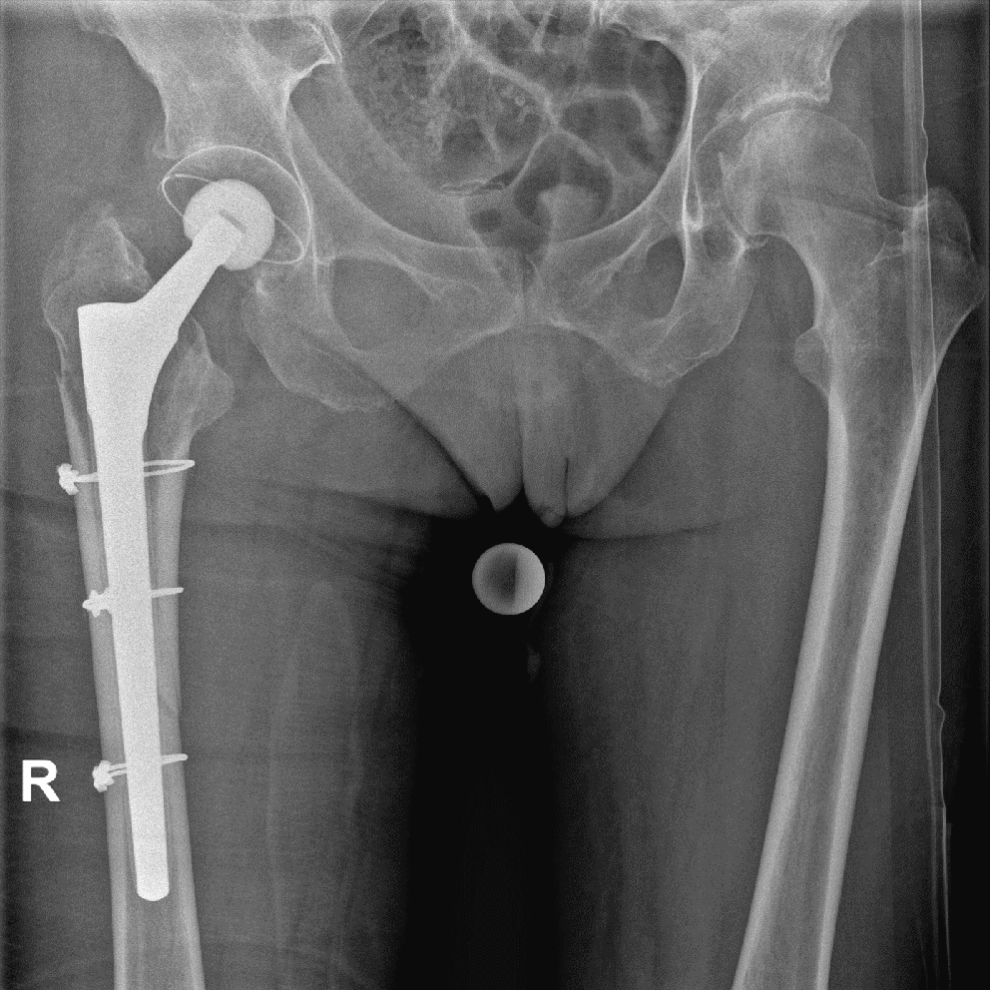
Postoperative Radiograph of the Vancouver B2 fracture depicted in Fig. 4 after revision arthroplasty with cerclage distal to the fracture and implantation of a Wagner non-cemented revision stem
